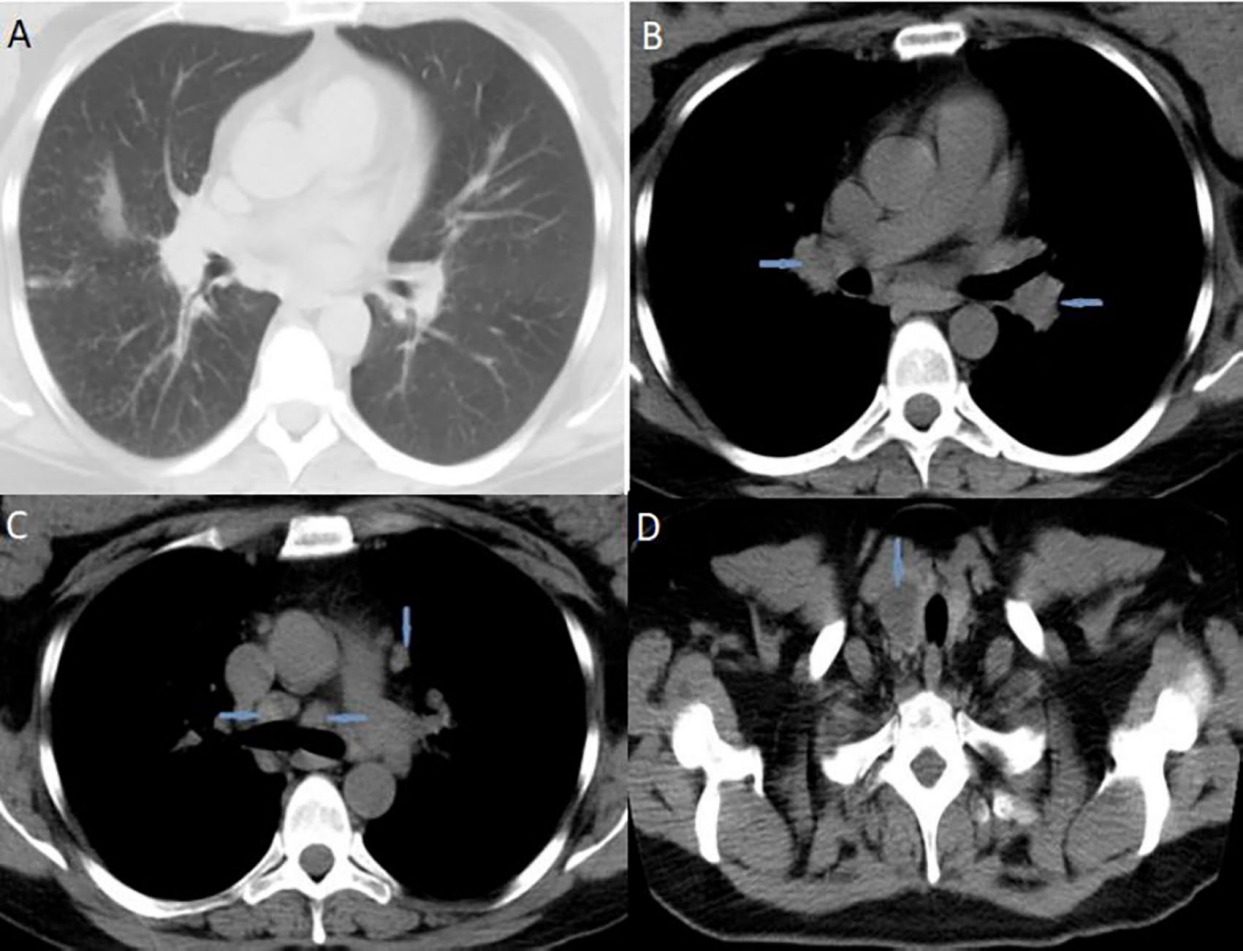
High-resolution computed tomography (HRCT) of the chest suggested pulmonary sarcoidosis: (A) micronodular lesions in pulmonary parenchyma; (B) hilar lymphadenopathy (blue arrows); (C) mediastinal lymphadenopathy (blue arrows). CT scans of the neck (D) indicated nodular mass inside the right thyroid lobe (blue arrow). HRCT, high-resolution computed tomography; CT, computed tomography.
